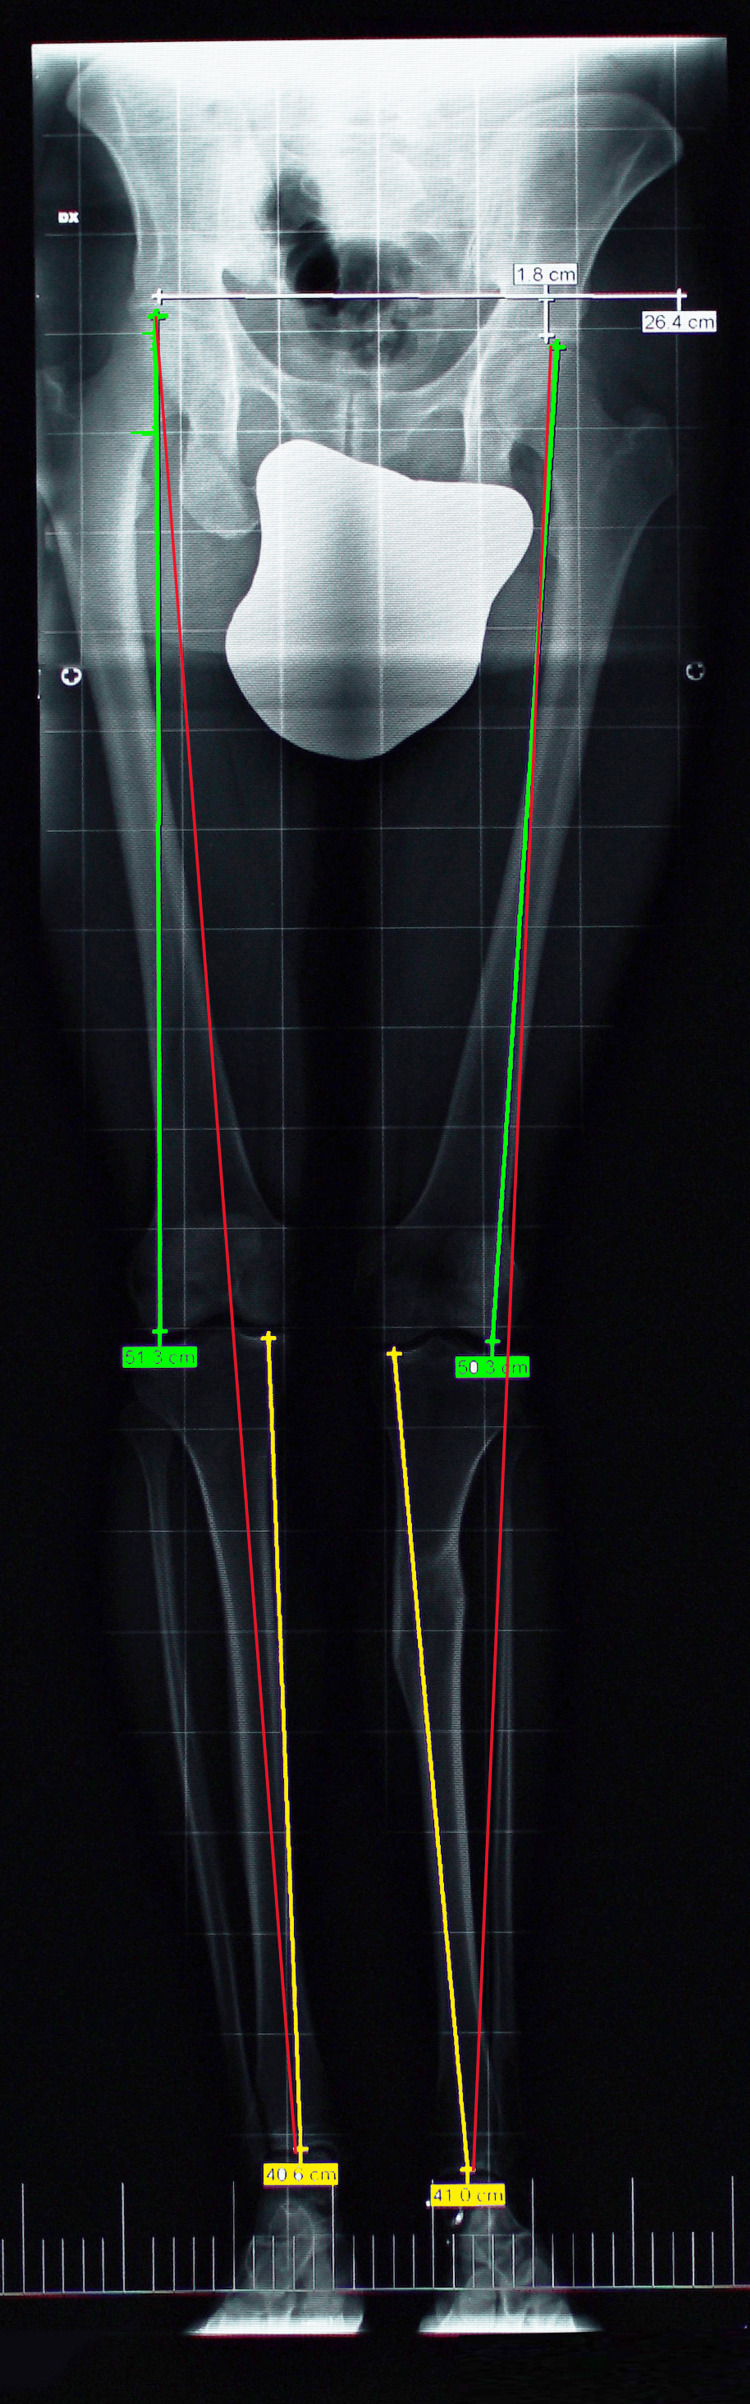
Anteroposterior radiograph in orthostatism of the lower limb (modified teleradiography) performed on X-rays by a computerized system. A patient with LLI due to a displacement fracture of the left tibia that altered the axis and length of the left limb. In addition to evaluating the length discrepancy of the entire limb (including the femur and the tibia), this imaging modality can be used to measure mechanical axis deviations and the angles of knee joint orientation, as proposed by Machen and Stevens [39]. LLI: leg length inequality Image Credit: Author Saverio Colonna
